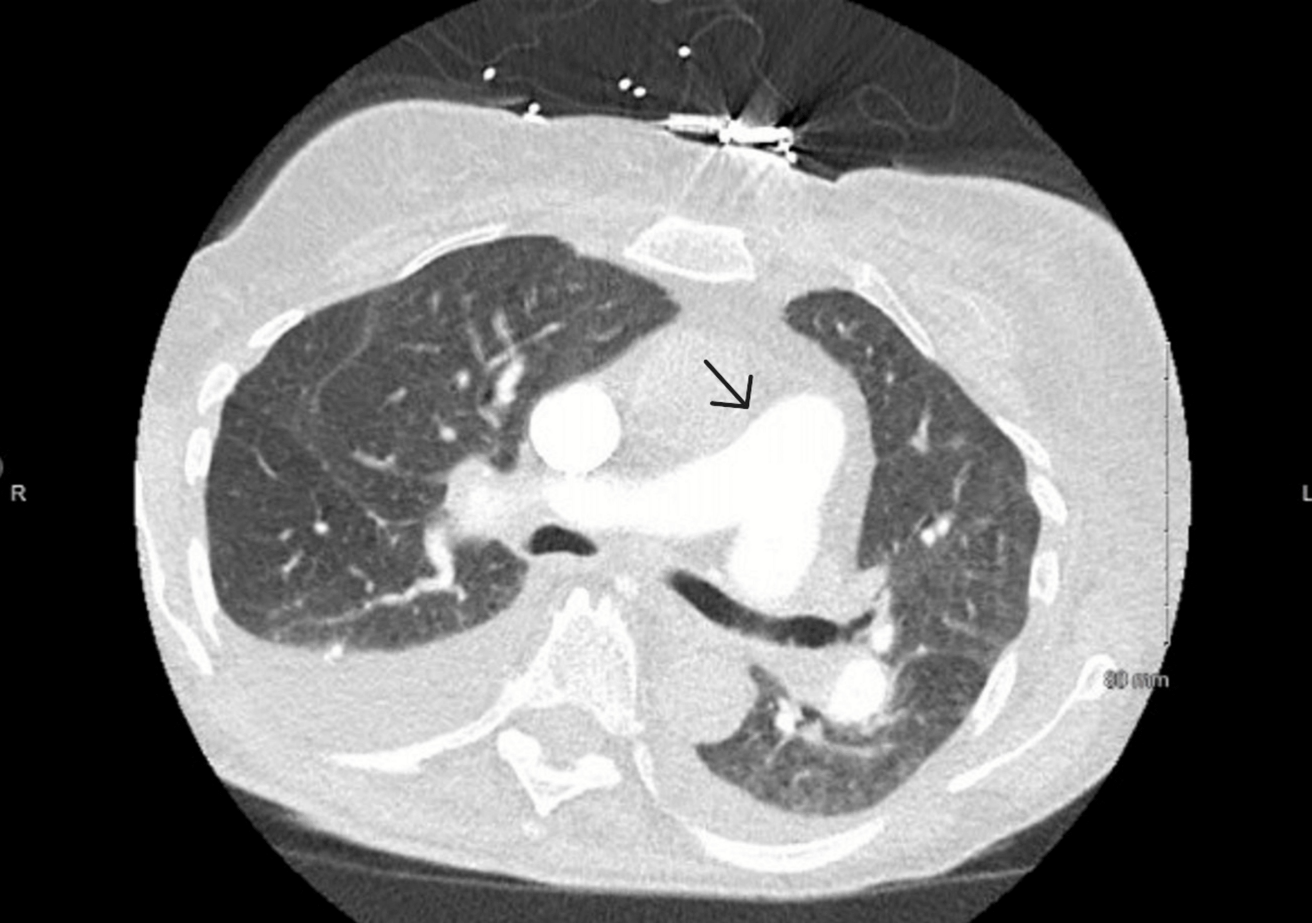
CT angiogram of the chest showed marked right heart and pulmonary artery enlargement (see arrow)
